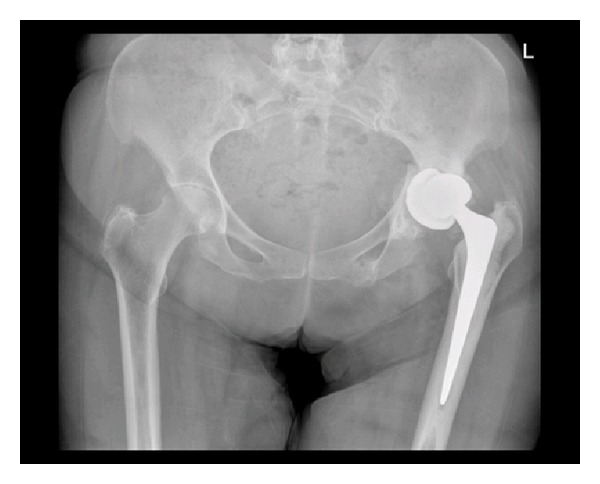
Pelvic radiograph taken after presentation with ongoing groin pain, showing fracture of the medial wall of the acetabulum with displacement of the acetabular component of the total hip replacement.
